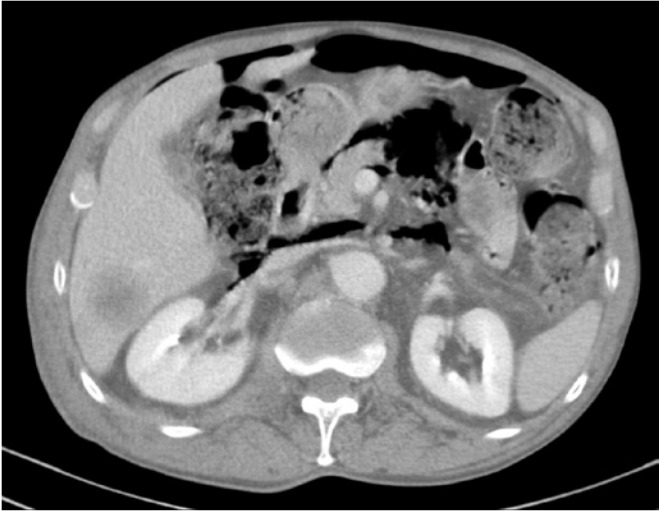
Abdominal computed tomography shows pneumatosis intestinalis
